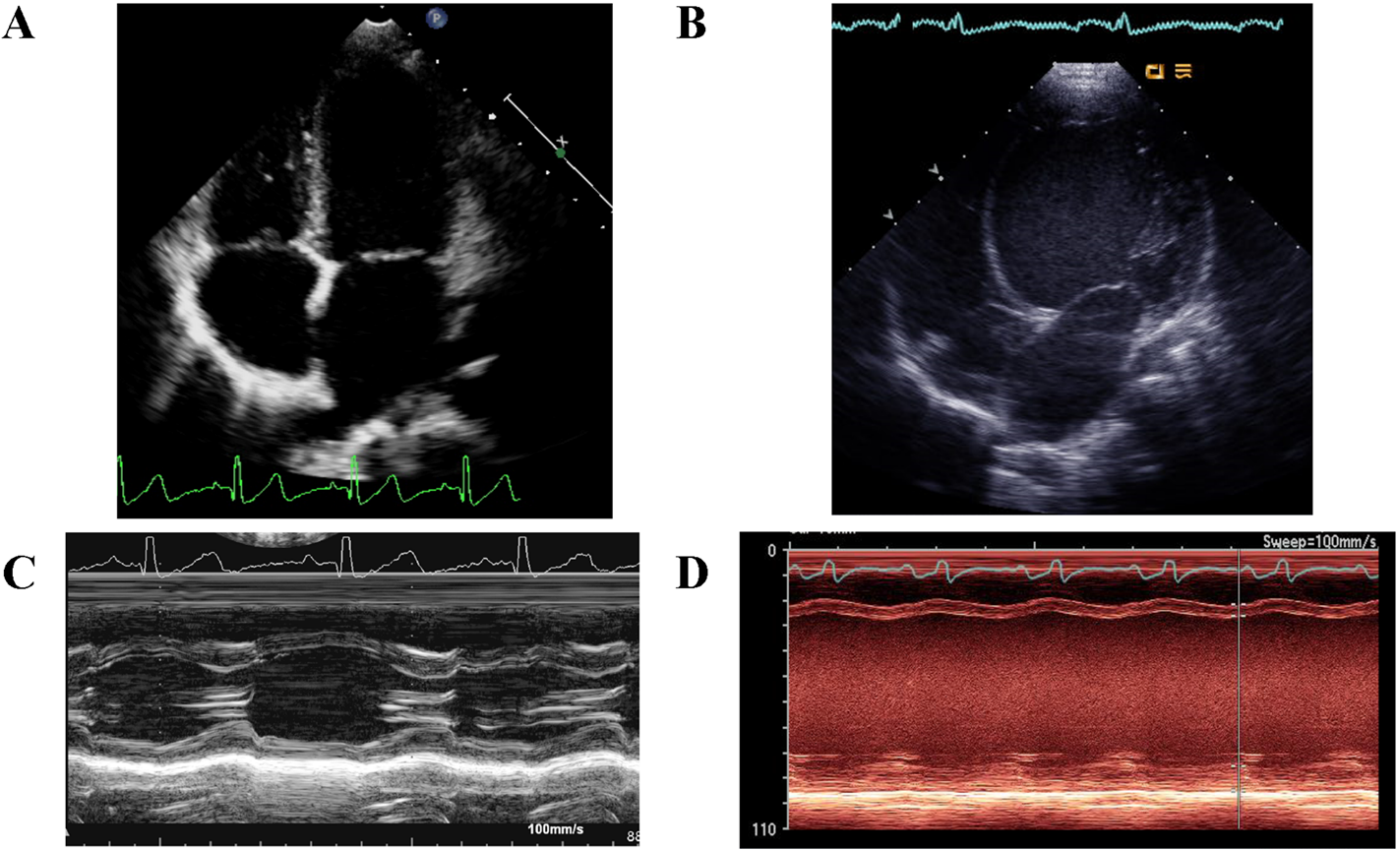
Transthoracic echocardiographic 4 chamber views (a, b) and m-mode at the level of the mitral valve (c, d) of the II-2 unaffected sibling (a, c) and the II-3 proband (b, d). A severely dilated left ventricle with poor systolic function is noted in the proband, while the unaffected sibling has normal cavity size and normal systolic function
